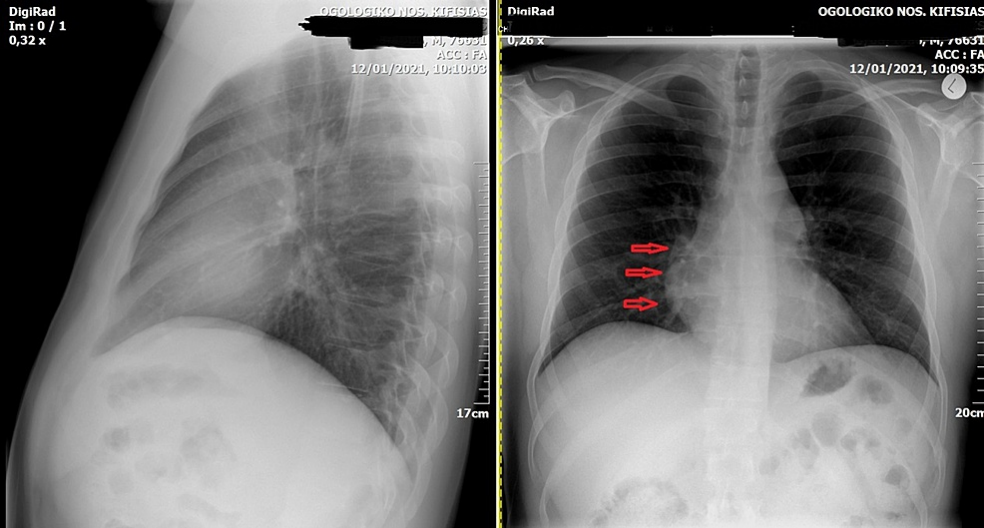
Lateral (left) and posteroanterior (right) chest radiograph showing the lesion (arrow) in the right cardio-phrenic angle.
